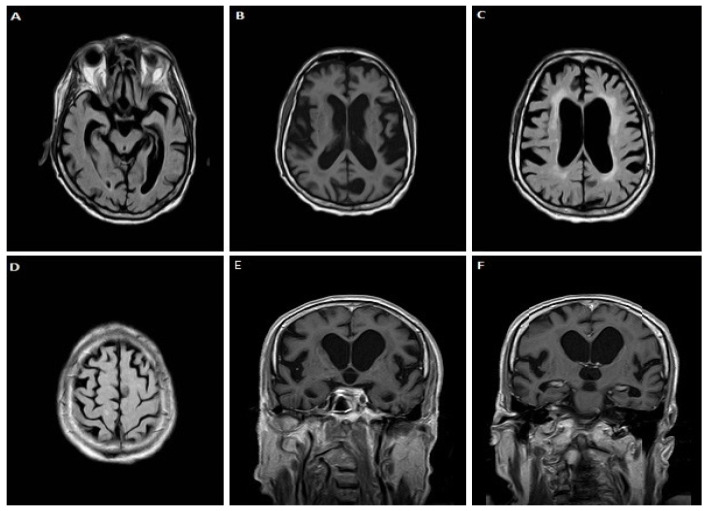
Brain MRI of case 3. FLAIR images (A,C,D) show severe cortical atrophy in frontal and temporal lobes. In axial (B) and coronal T1 section (E,F) atrophy of the perisylvian and parietal areas is also evident.
